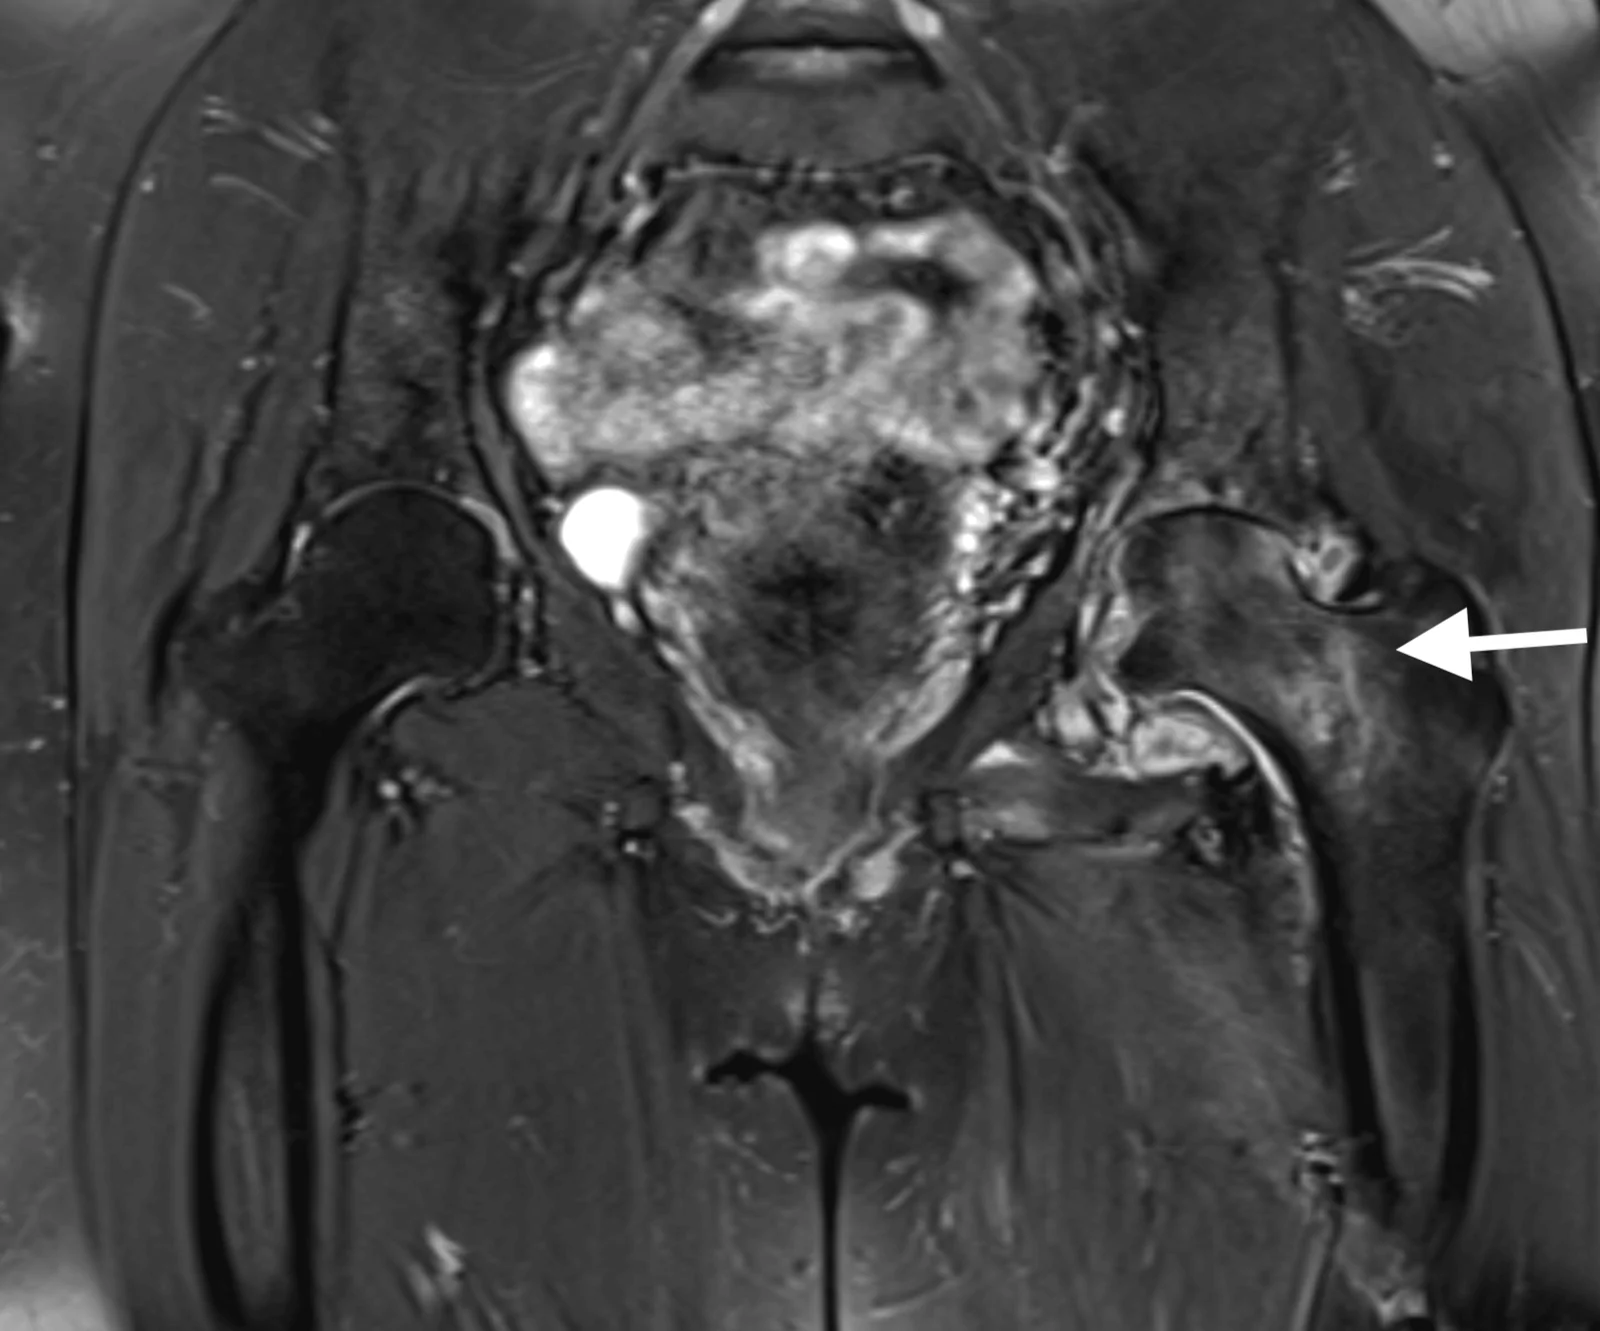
Coronal fat-suppressed T2-weighted MRI of the pelvis showing left femoral osteomyelitis with intraosseous abscess formation (arrow) and joint effusion. The image shows a hyperintense area in the left femoral neck corresponding to osteomyelitis with intraosseous abscess formation, as indicated by the arrow. The abscess measures 3.5 × 2.2 × 1.3 cm in the craniocaudal, transverse, and anteroposterior dimensions, respectively. A reactive joint effusion is also present.
